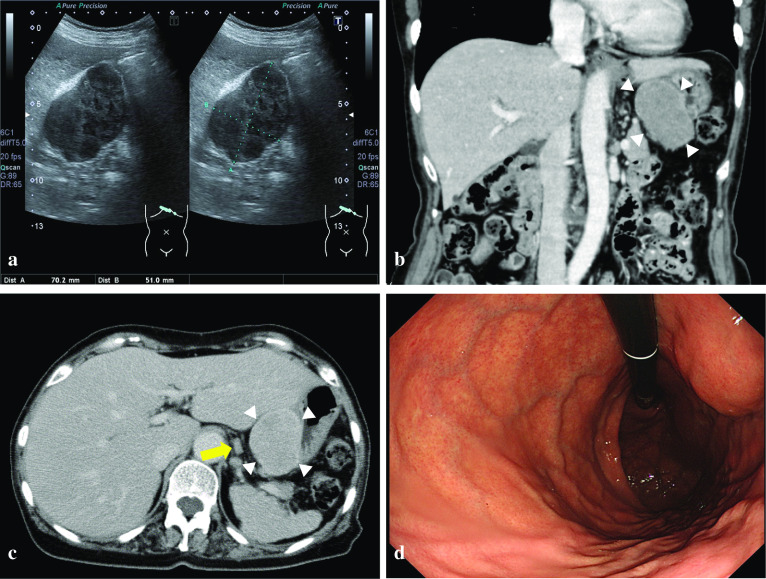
a Abdominal ultrasonography revealing a well-circumscribed hypoechoic mass, contiguous with the proper muscle layer, approximately 70 mm in size, located at the middle stomach on the lesser curve. b, c Contrast-enhanced CT of the abdomen showing an extraluminal tumor with heterogeneous enhancement at the middle stomach on the lesser curve (white arrowheads), accompanied with one swollen lymph node approximately 10 mm in size (yellow arrow) and several small lymph nodes in the perigastric region. d Endoscopy showing compression of the anterior wall of the gastric body along with normal mucosa
